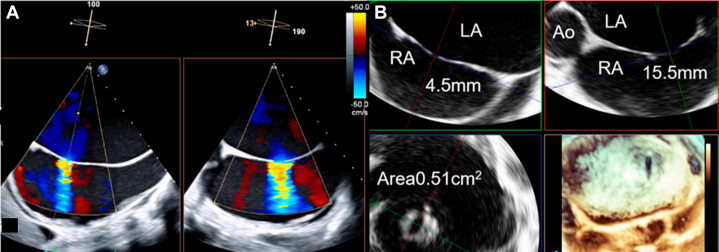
Baseline iASD Assessment Immediately Post-M-TEER Intraprocedural transesophageal echocardiography at the conclusion of the procedure. (A) Two-dimensional (2D) color Doppler showing the initial left-to-right shunt after 24-F sheath withdrawal. (B) 3D quantification analysis demonstrating an iatrogenic atrial septal defect (iASD) orifice area of 0.51 cm^2^ (major/minor axis: 15.5/4.5 mm). LA = left atrium; M-TEER = mitral transcatheter edge-to-edge repair; RA = right atrium.
